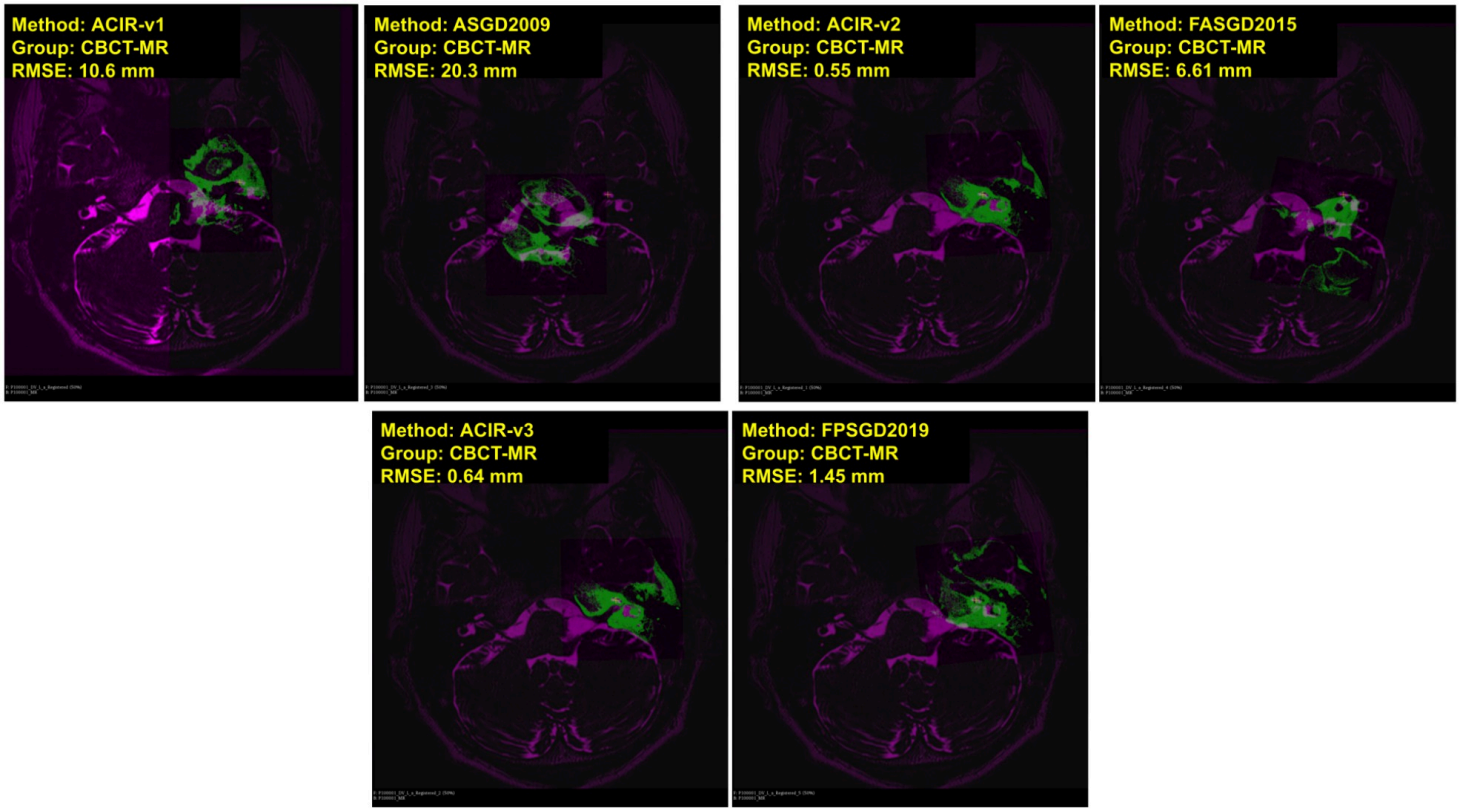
Sample results of registration CBCT to MR. The figure shows the axial views with image types, methods, and RMSE information. Magenta represents the fixed image and green represents the moving image.
